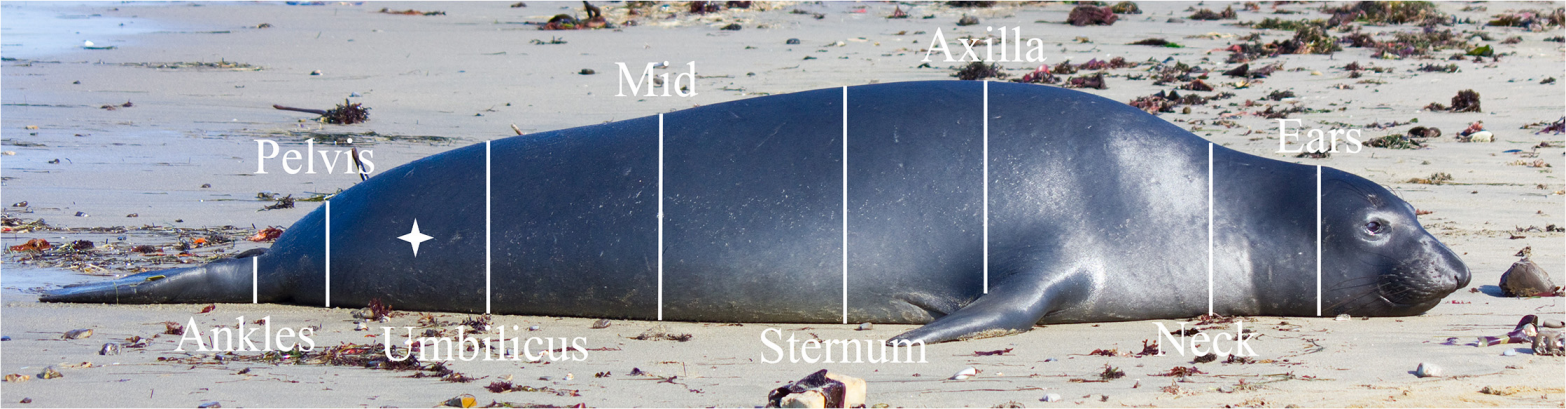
Morphometric measurement locations. Locations of width, height, girth, and curvilinear measurements. Dorsal and lateral ultrasound images were also taken at all locations except ears and ankles. Star indicates location of blubber biopsy. Photo by Patrick Robinson under NMFS Permit 14636.
